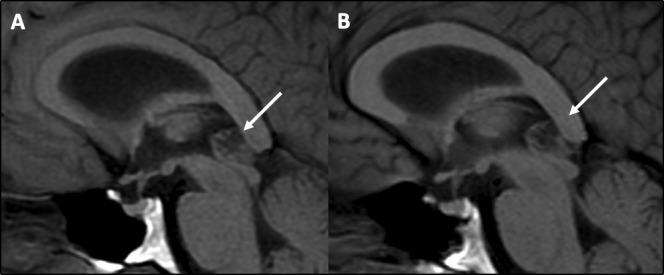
MR scan of brain showing no change in size, shape or appearance on sagittal T1-weighted MRI sequences of an atypical pineal cyst (arrows) in 2016 (A) and 2020 (B).
